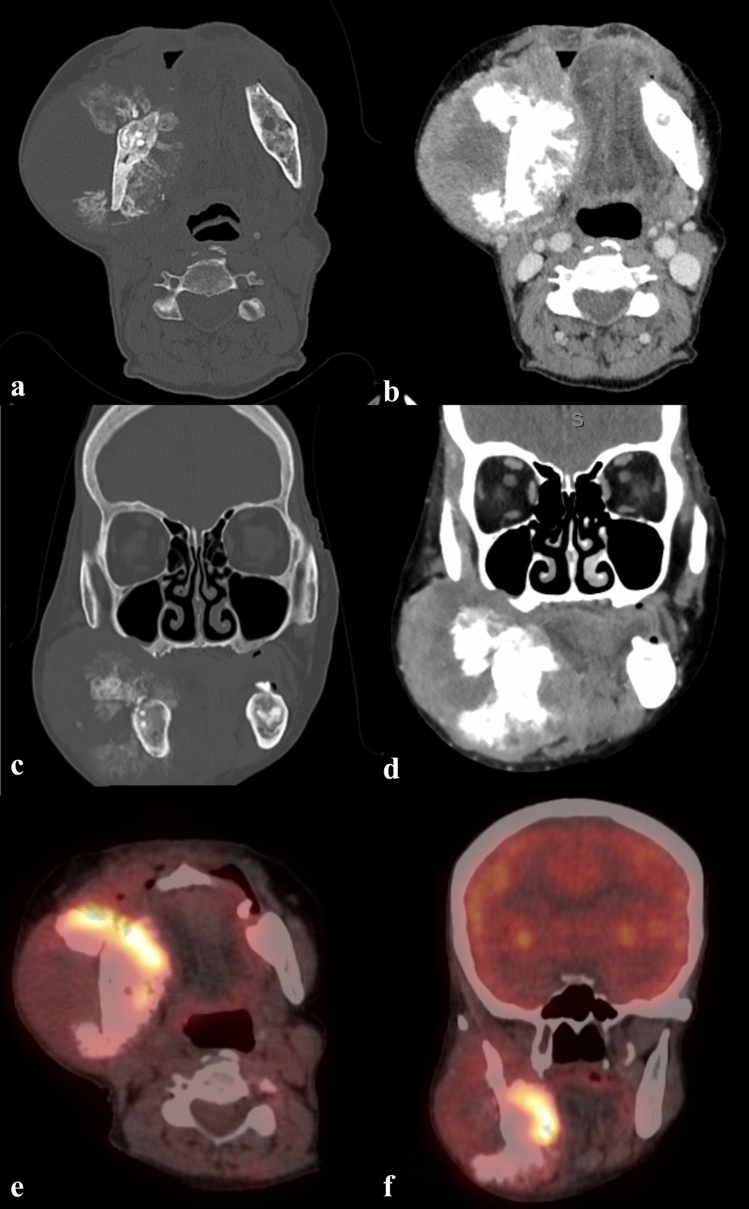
a CT axial slice, b CT axial slice with contrast, c CT coronal slice, d CT coronal slice with contras. a–d: The sclerotic bone lesion has a sunburst appearance arising from the inner cortex of the mandible with associated heterogeneously enhancing mass which extends to the masticator, buccal and carotid spaces. There is erosion of the posterior wall of the right maxillary sinus. Mass effect on the tongue displacing the tongue to the left. e PET scan—axial, f PET scan—coronal. e–f: Increased uptake noted in the anterior and medial aspect of the lesion
